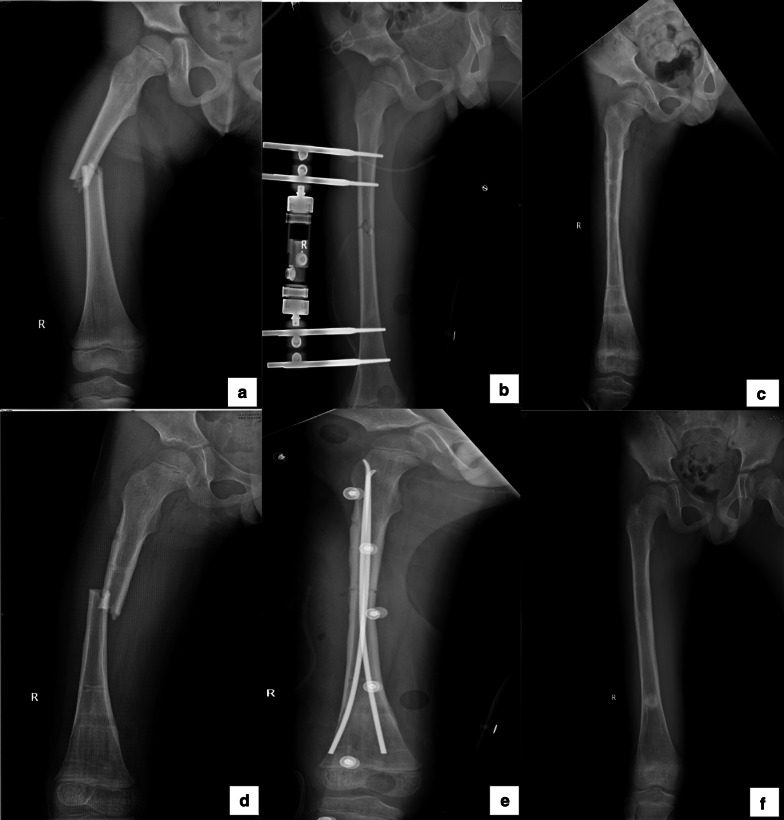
Radiograph showing the right femoral shaft fracture in a 6-year-old male patient. a Initial fracture; b Fracture treated with external fixation (EF); c Removal of EF after 8 months; d At the fourth week after the fixation removal, refracture, unfortunately, happened due to a fall; e Refracture treated with an elastic intramedullary nail; f Removal of the fixator after 11 months. No refracture occurred within 1 year
